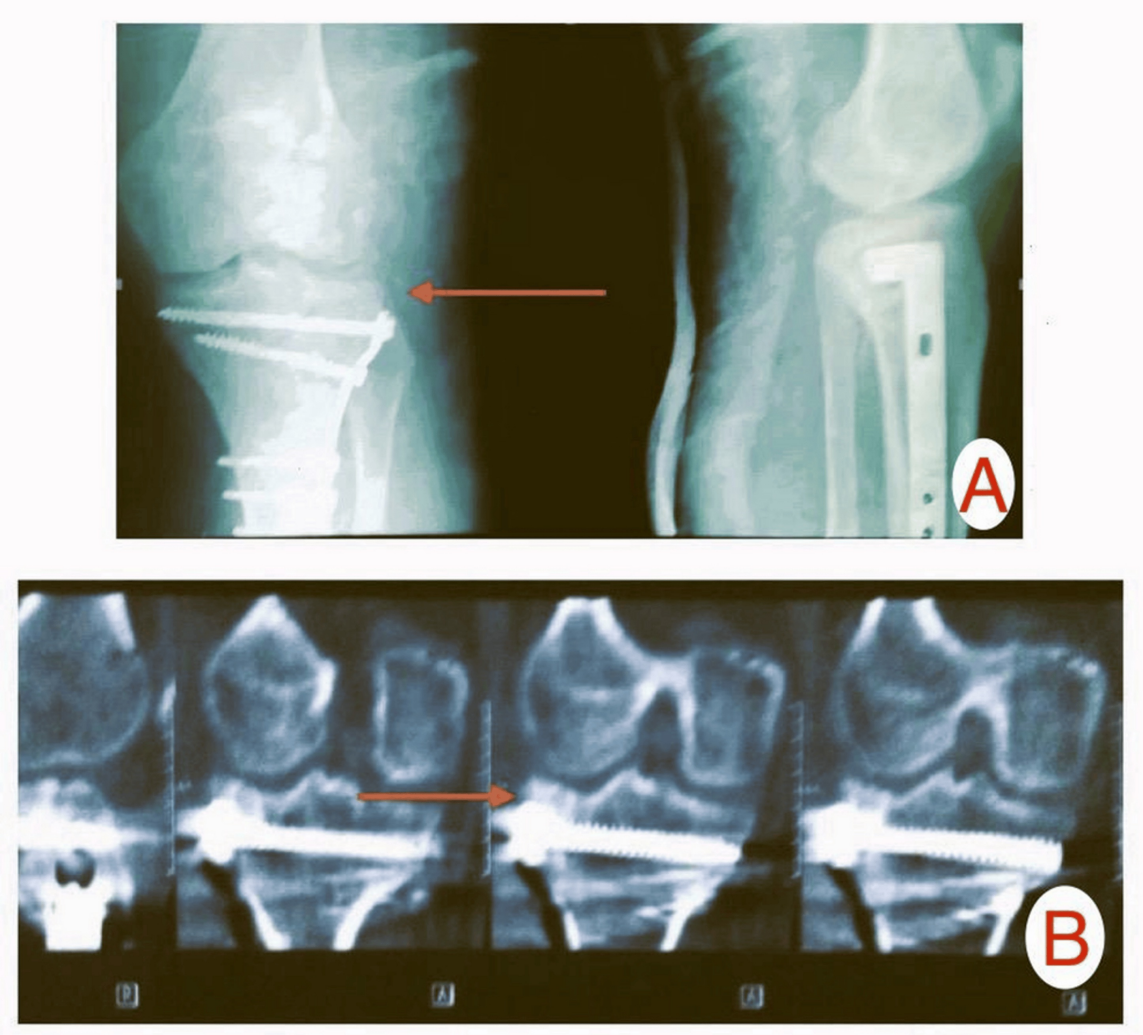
Immediate postoperative images of Patient B: (A) X-ray showing a reduced tibial plateau fracture, (B) CT scan showing reduction and elevation of the depressed fracture and restoration of articular congruity
